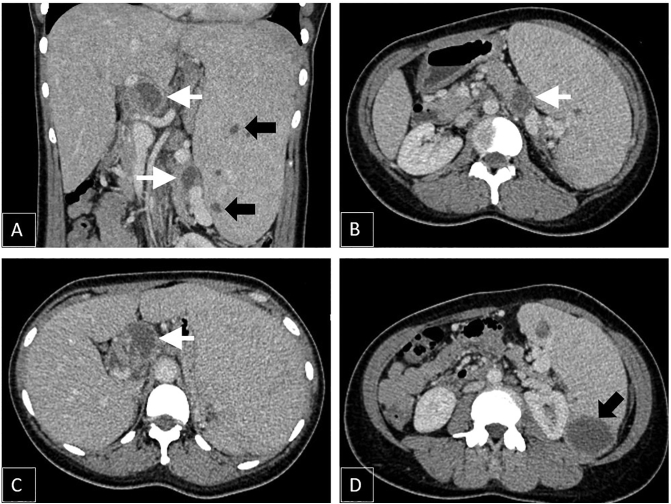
Contrast-enhanced CT (axial and coronal) images A-D showing multiple rim-enhanced cystic lesions within spleen parenchyma (black arrow) with multiple lymph node enlargement with central necrosis (white arrow).
